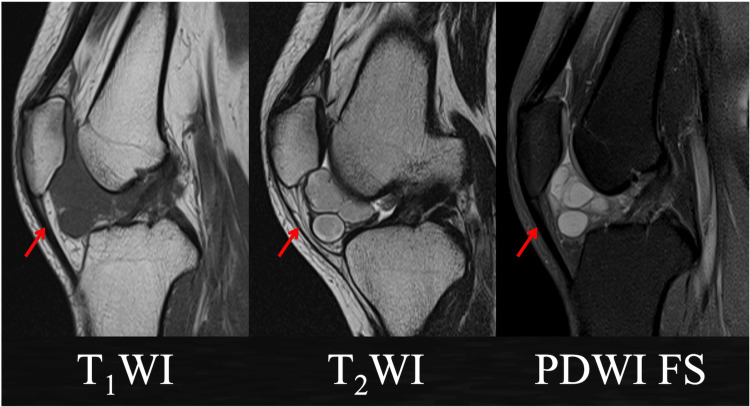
Magnetic resonance images of the knee A multifocal mass in the infrapatellar fat pad was observed, with a low signal on T1WI, a high signal in the periphery, and an equally high signal on T2WI, with no fat suppression and no damage to the articular cartilage, meniscus, or ligaments.
